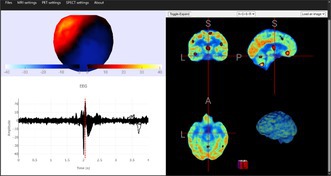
FIGURE 1 Web‐app interface with EEG (right) and MRI/PET imaging (left). Time navigation is performed in the EEG panel, slice and view selection in the imaging panel, and a central menu bar controls independent opacity and thresholding of each volume.
